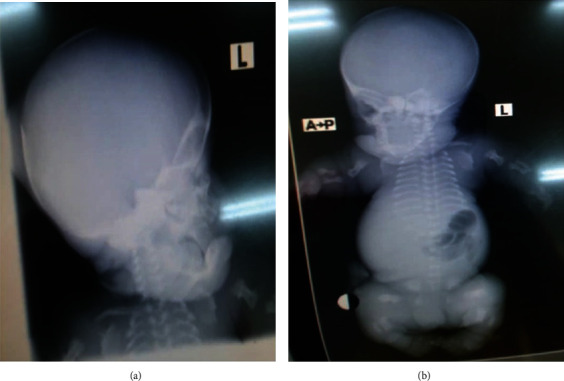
Postmortem radiograph of the skull. (a) Showing macrocephaly, frontal bossing, and flattening nasal bridge. (b) Showing proximal portions of the long limbs are small, giving a rhizomelic appearance. The humeri and femora have typical “telephone handle” bowing with metaphyseal flaring. The iliac bones are hypoplastic. Short horizontal ribs, narrow chest, and small scapulae.
